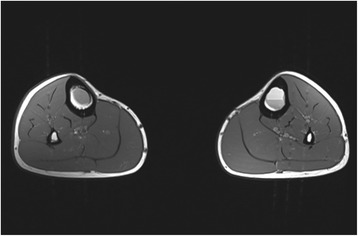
T1-weighted transversal MRI of bilateral simple bone cysts confirming its fluid content. On the left side, fluid-fluid levels created by the different densities of the cyst fluid caused by the settling of red blood cells are existent. A septation of the cyst or bloody content is more characteristic of a fractured UBC but can be interpreted as previous (repetitive) injury to the bone in this case
